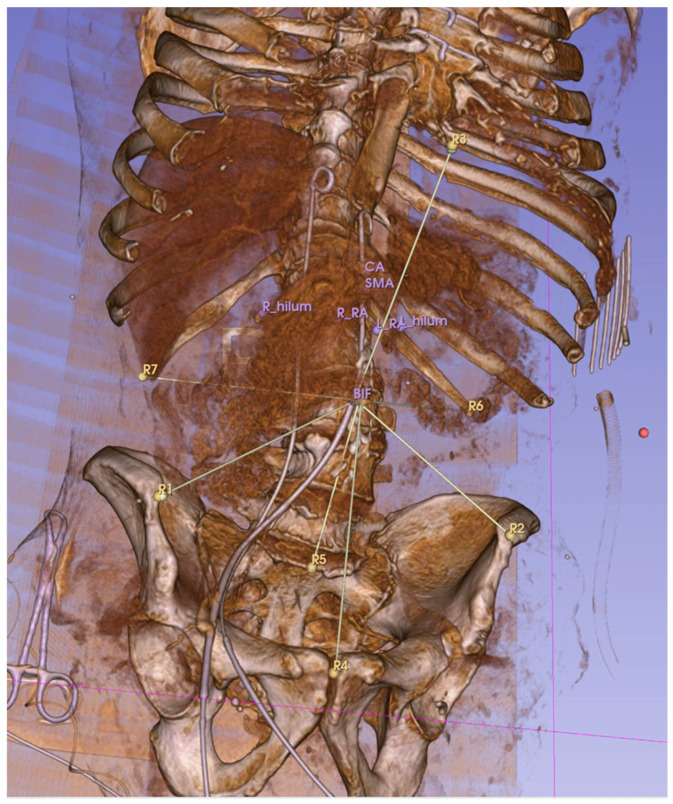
Euclidean distances between reference points (R1–R7) and target anatomical locations were measured. This 3D, reconstructed CT image shows the measurement lines connecting the aortic bifurcation to the reference landmarks R1–7. The distal tip of the sternum (R3 here) was later excluded due to its broad movement associated with the abdominal pressure increase.
